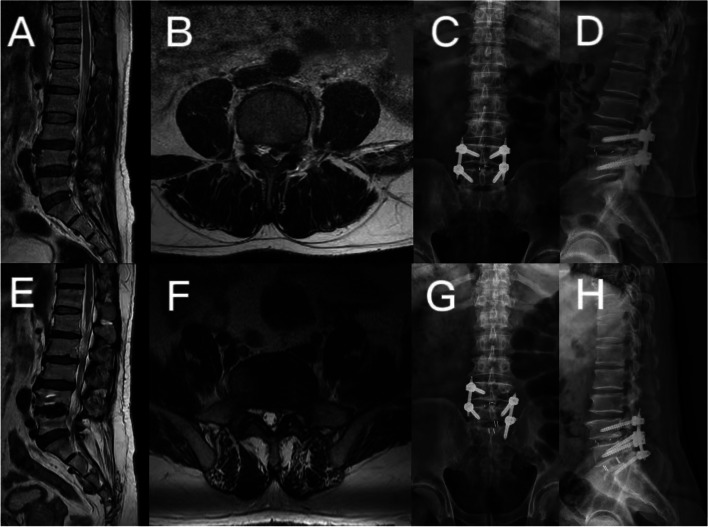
A 54-year-old male, with L4/5 lumbar disc herniation (A and B), MIS-TLIF was performed on L4/5 in November 2012 (C and D). In August 2021, the patient developed numbness and pain in the left leg. MRI showed that the L5/S1 disc was herniated and compress the left nerve root (E and F). His diagnosis of ASD and MIS-TLIF was performed by MRI at L5/S1 (G and H)
